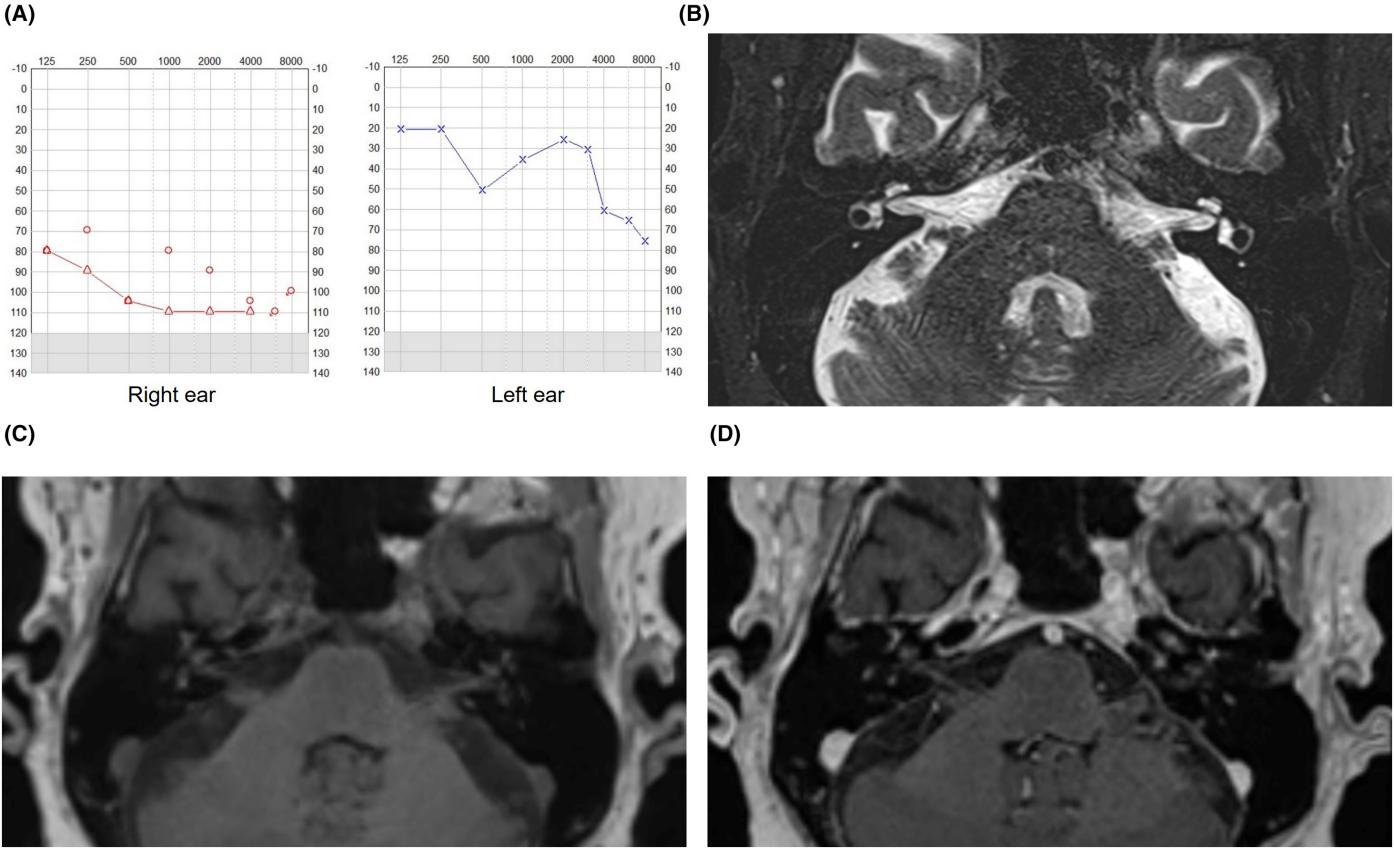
(A) Tone audiogram 6 months after debut of inner ear symptoms. (B) T2‐weighted MRI imaging revealing normalization of perilymph signal in the cochlea. (C, D) T1‐weighted MRI imaging before and after intravenous gadolinium contrast medium showing normalization of perilymph signal in the cochlea and in the vestibular apparatus. No sign of pathological contrast enhancement in the labyrinth.
